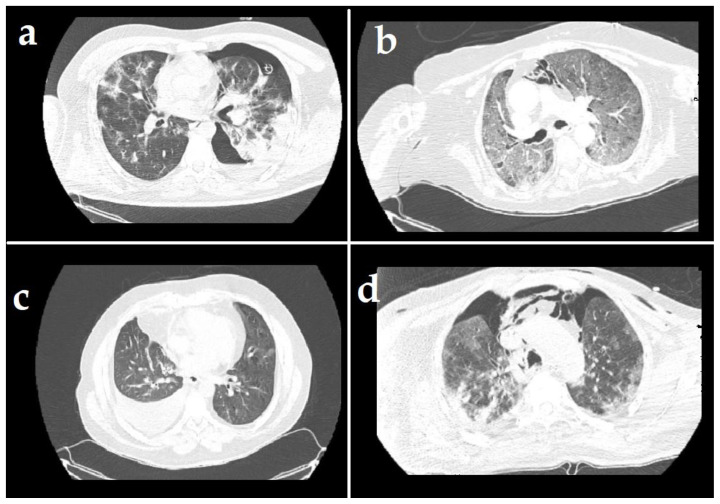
a) Thorax CT shows left-sided pneumothorax in a patient with COVID-19 pneumonia; b) Coexistence of pneumomediastinum and diffuse ground glass opacities are seen in tomography scan; c) Tomographic view of right-sided pleural effusion and mild COVID-19 pneumonia; d) Thorax CT scan shows pneumomediastinum and bilateral pneumothorax.
